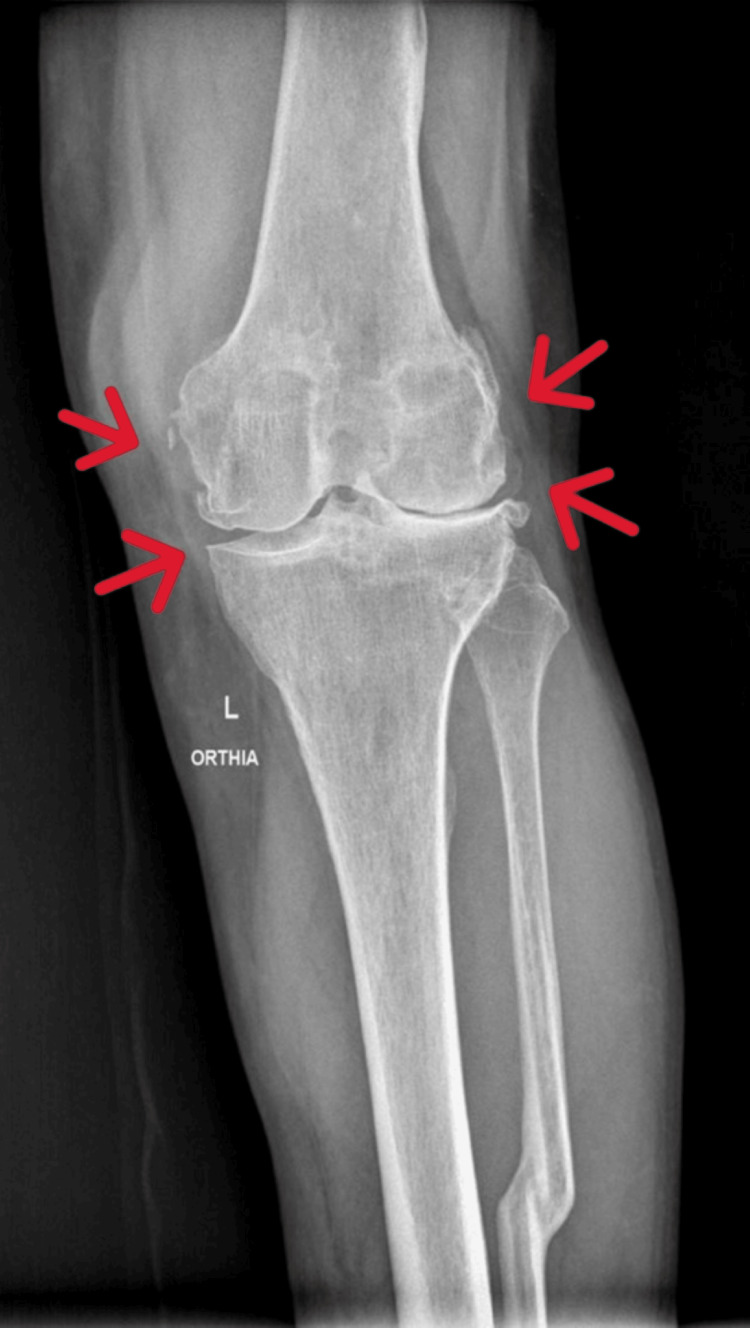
Preoperative Anteroposterior X-ray of the Left Knee (Weight-Bearing, Arrows)
